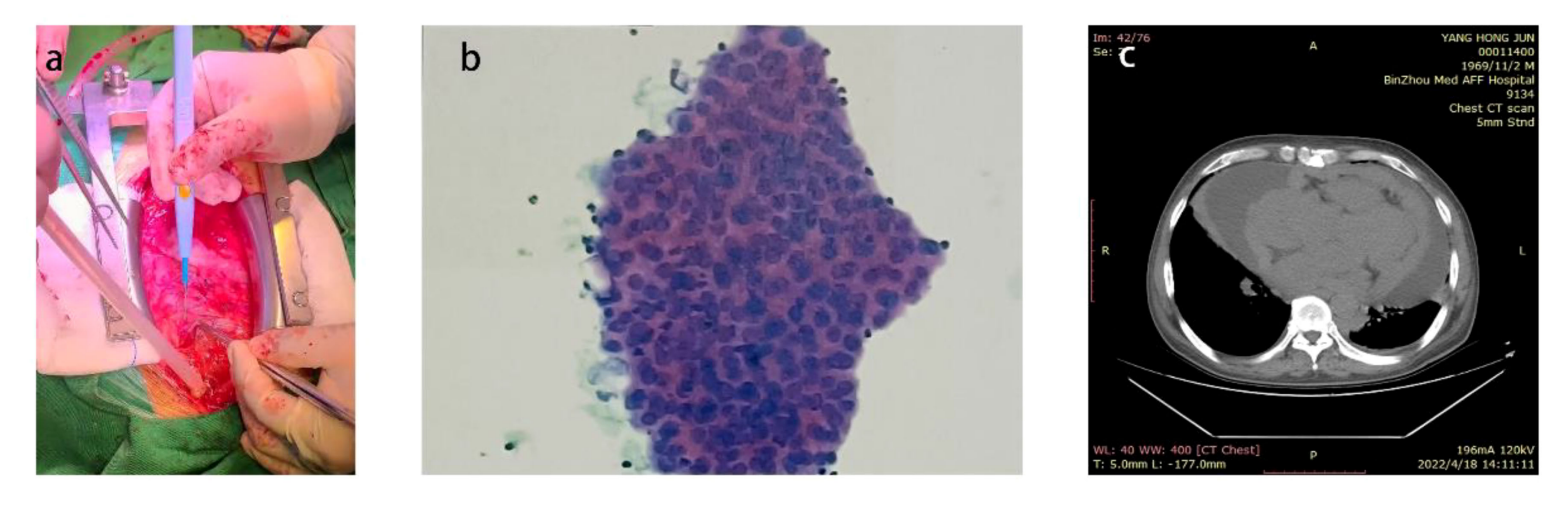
(A) The patient presented with a significant volume of pericardial effusion within the pericardial cavity. (B) Pathological results of pericardial effusion (C) Preoperative chest CT prompted a large number of pericardial effusion.
